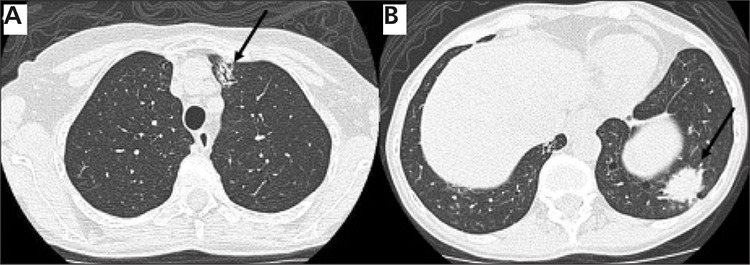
Axial images of a diagnostic CT scan on lung windowsettings demonstrate a spiculated 2x1.2 cm ill-defined opacity(arrow) in the anterior left upper lobe, and 2.6x2.2 cm opacity(arrow) in the posterior left lower lobe
